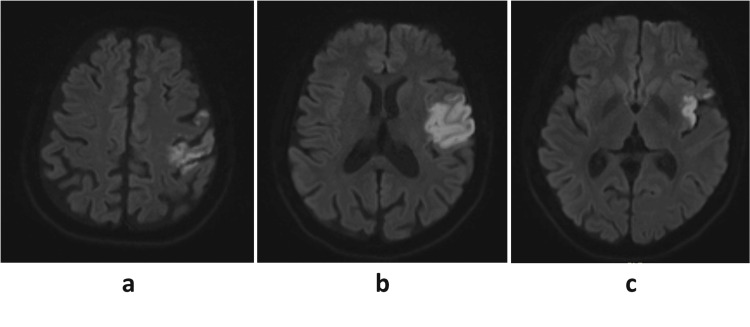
Axial magnetic resonance imaging (DWI) Axial magnetic resonance shows restricted diffusion along the left middle cerebral artery territory involving the (a & c) insula and (b) frontoparietal lobe.
